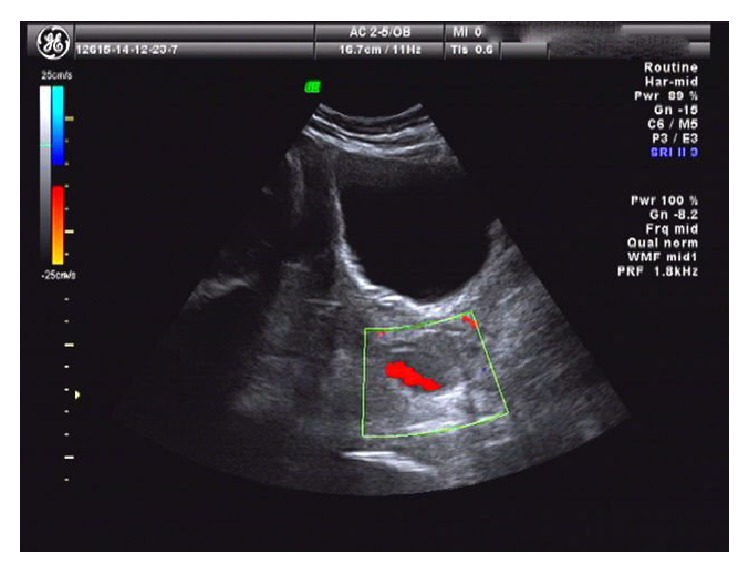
Sonographic findings showing unilocular cysts containing hyperechoic fluid and irregular wall with some blood flow signals.
